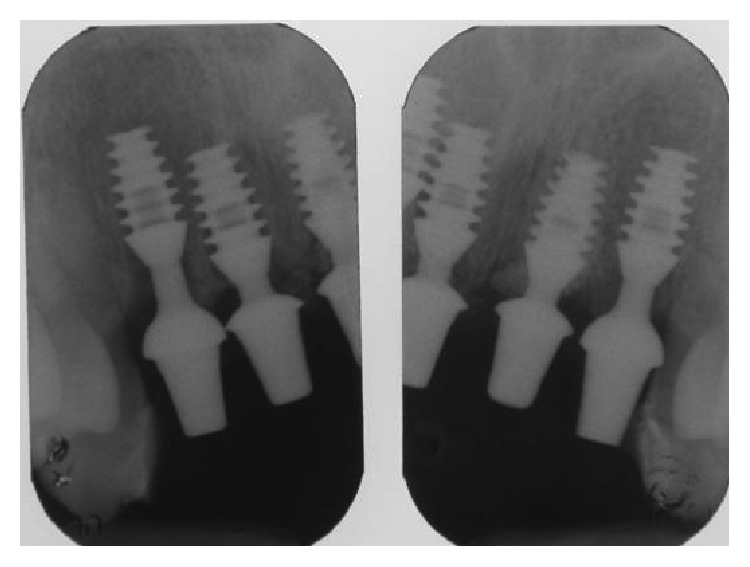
Postsurgical X-ray showing implants after surgery with provisional abutments inserted.
